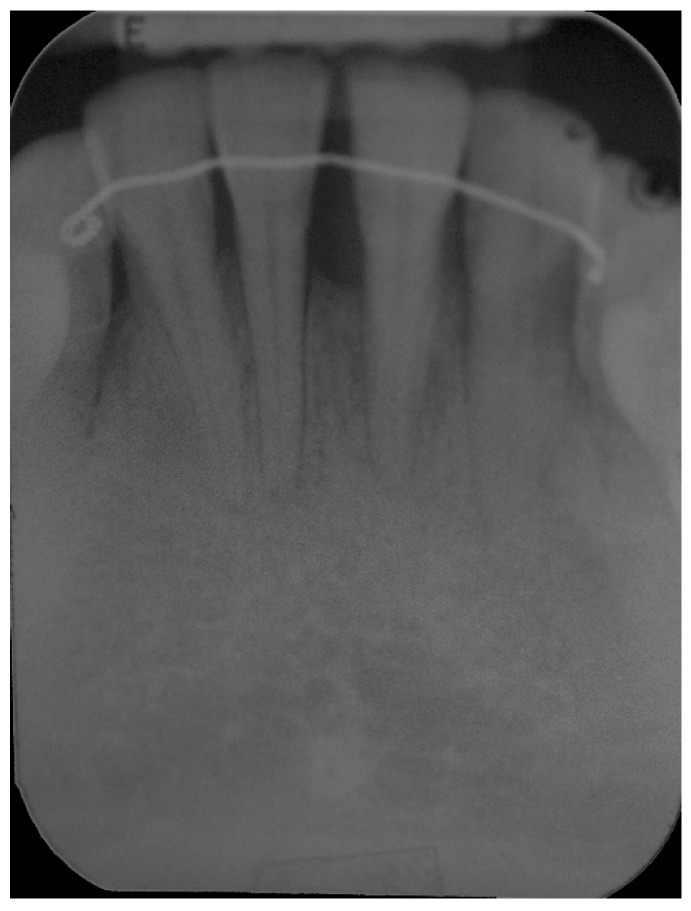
One-year post-operative radiograph, improvement in bone support, especially around the central left incisor is evident, compared to the pre-operative x-ray, the bone defect around the left central incisor has largely been reduced.
